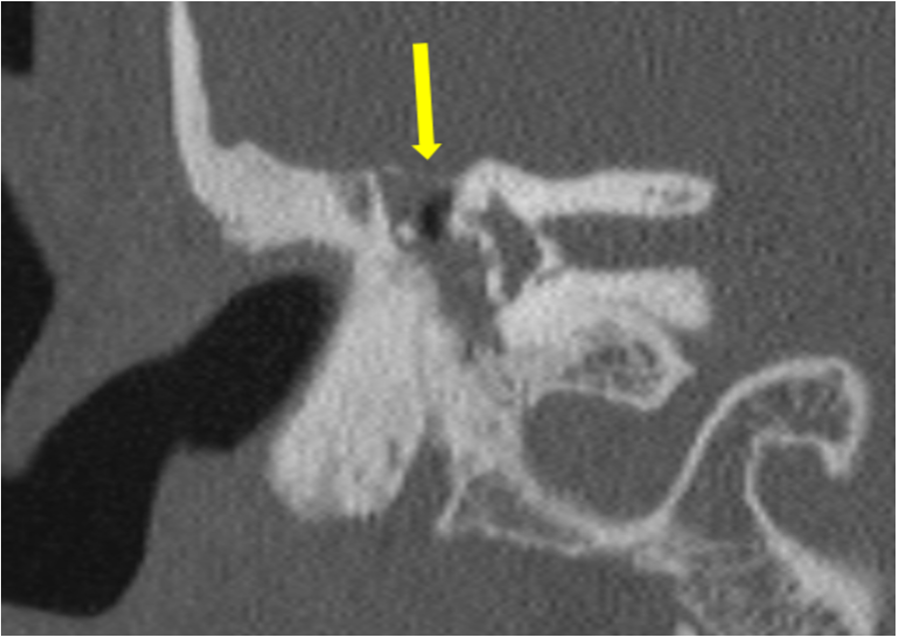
Coronal CT image showing erosion with focal defects in tegmen tympani (arrow).
